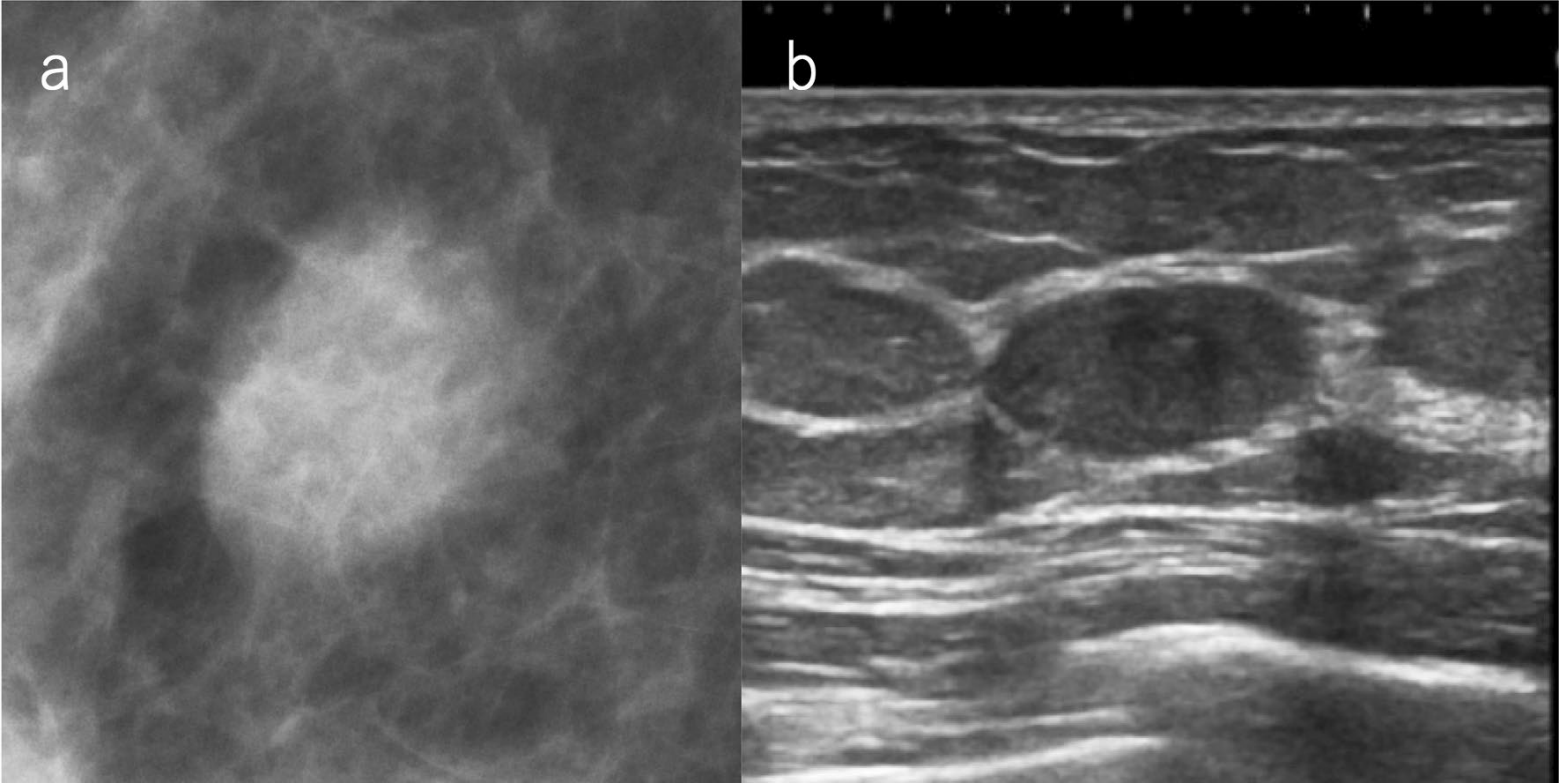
A case of circumscribed mass on mammography. a Mammography: oval mass with margin mostly obscured but also circumscribed, SMC 3. b Ultrasound: oval, circumscribed, and parallel to the skin; classically fibroadenoma, SUC 2. The overall assessment category: SC 2 (*SMC* screening mammography category, *SUC* screening ultrasound category, *SC* screening category)
